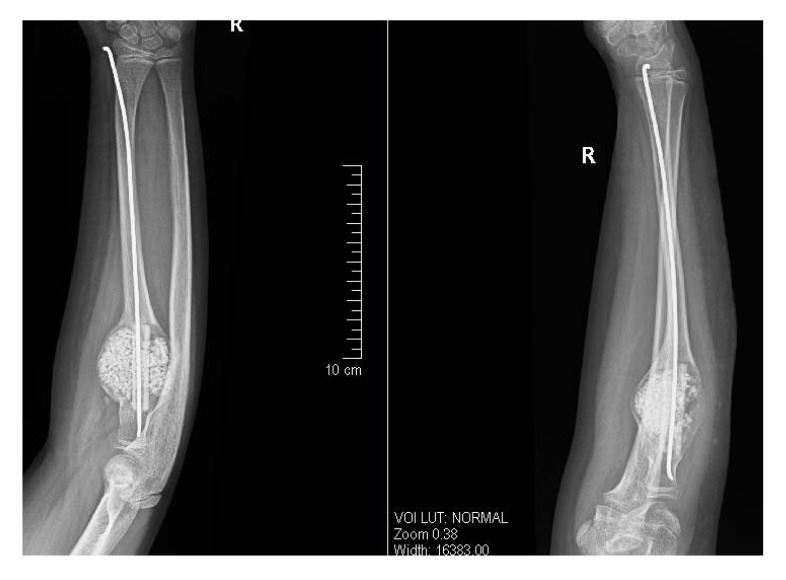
Immediate post op radiograph showing completely curetted cystic bony lesion with filled allograft granules, bridging fibular strut graft and transfixing intra-medullary titanium elastic nail insitu.
